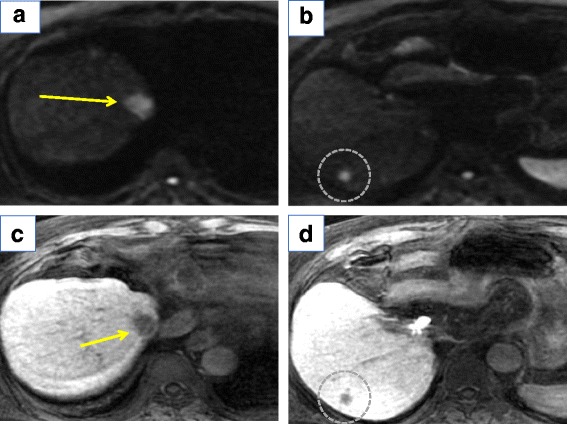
Gd-EOB-DTPA-enhanced MRI shows two lesions, one each in segments 7 and 8. a, b Diffusion-weighted imaging showing high-intensity lesions. c, d Gd-EOB-DTPA-enhanced MRI shows low-intensity lesions in the hepatocellular phase
